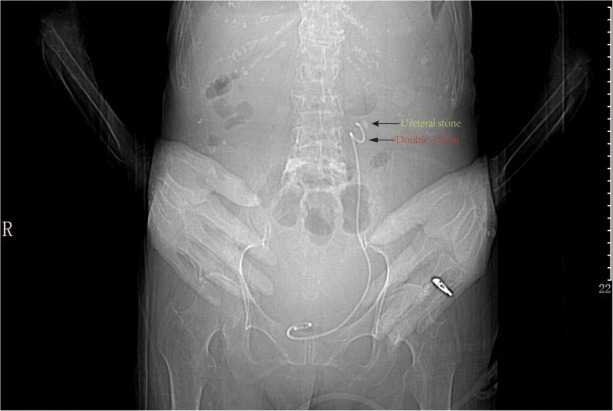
KUB radiography taken on the 1st postoperative day.
